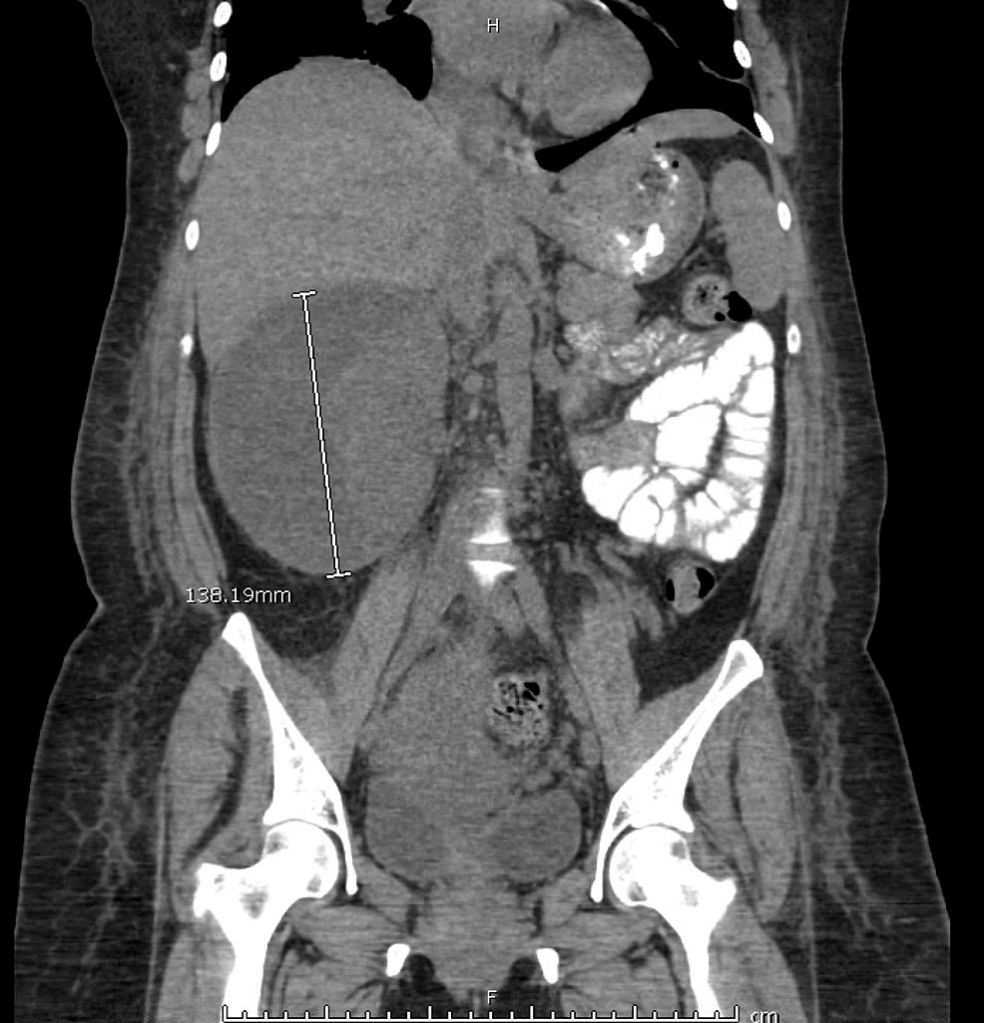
Coronal CT image of the renal abscess
